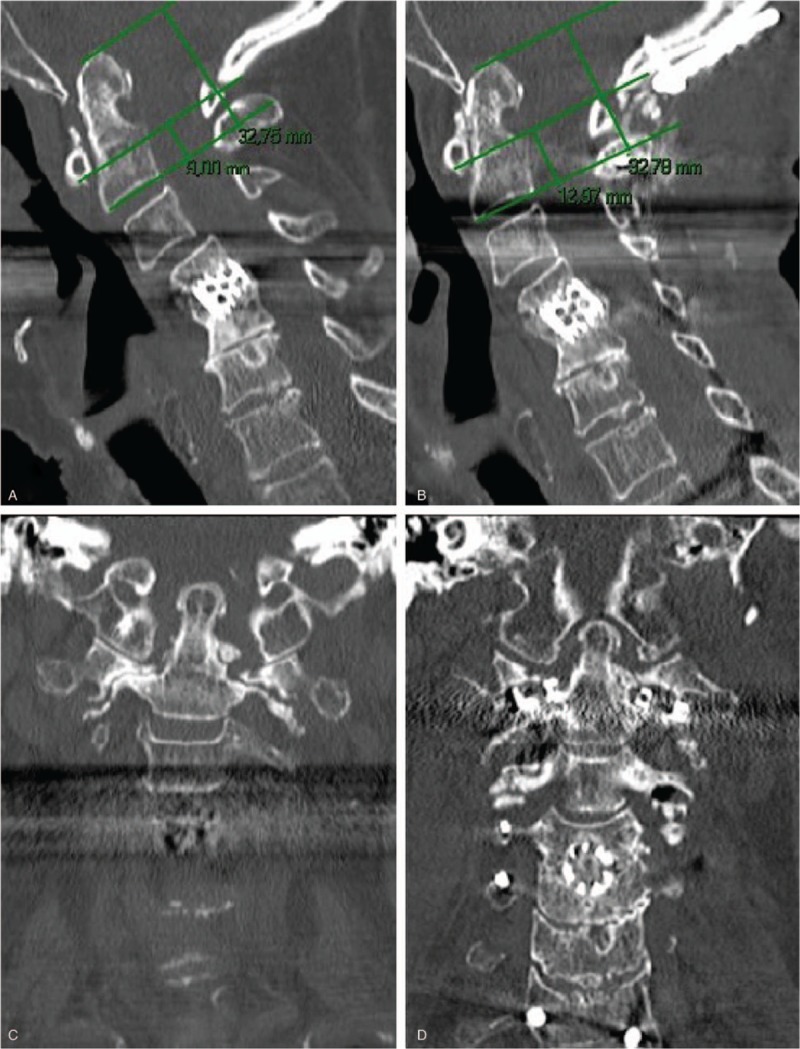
Images from case 2. (A) Preoperative sagittal CT image. The lines required to measure VAAI are shown. VAAI = 0.27. (B) Preoperative coronal CT image. (C) Postoperative sagittal CT image. The lines required to measure VAAI are shown. VAAI = 0.402. (D) Postoperative coronal CT image. The C1–C2 facet spacers are inserted bilaterally. CT = computed tomography, VAAI = vertical atlantoaxial index.
